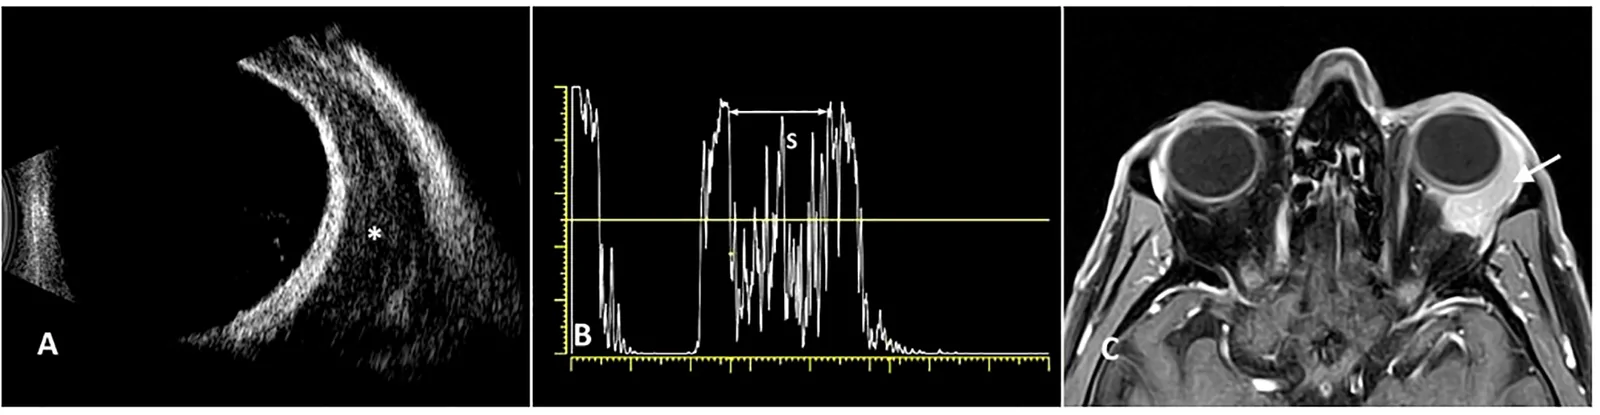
Orbital Lymphoma. (A) Longitudinal B-scan shows a well-outlined low-medium reflective lesion (asterisk) molding to the globe. (B) Corresponding A-scan displays mainly low-medium amplitude spikes (double arrow) and a distinct high amplitude spike consistent with internal septa *(S*). (C) MRI axial shows a large lymphoma (arrow) on the lateral region, molding around the globe.
